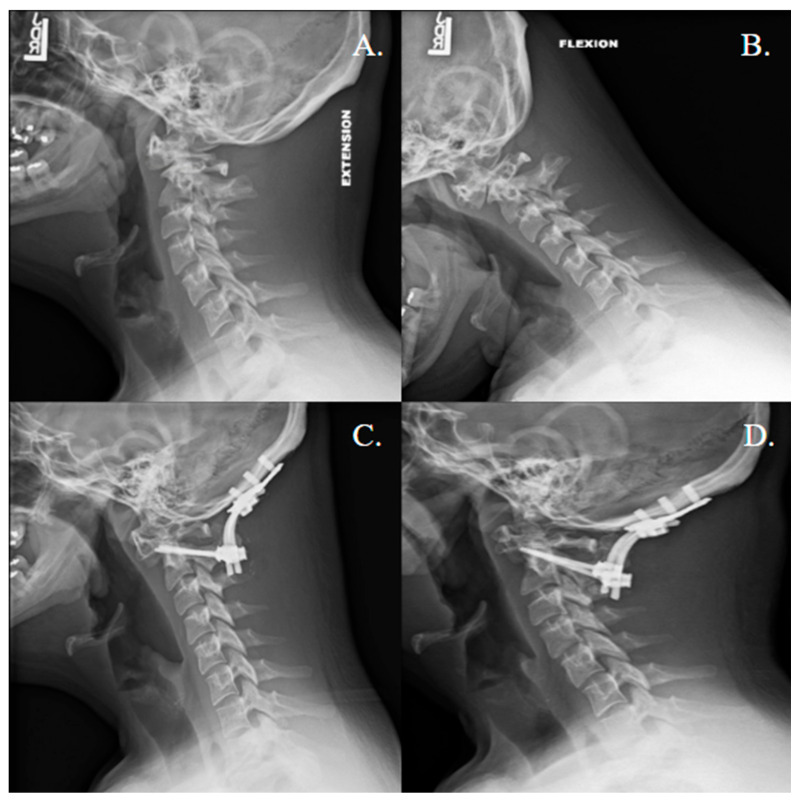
Atlantoaxial instability in Down syndrome: dynamic instability, construct, and follow-up. (A) Pre-operative lateral extension radiograph with ADI measuring 3 mm. (B) Pre-operative lateral flexion radiograph with anterior ADI measuring 6 mm, demonstrating dynamic C1–2 instability. (C) Immediate post-operative lateral radiograph demonstrating C1–2 instrumented fusion with appropriate hardware position and reduction in the ADI. (D) One-year post-operative lateral radiograph showing maintained reduction, no hardware lucency, consistent with fusion. Abbreviations: ADI: anterior antlanto dental interval; LDR: low dose reconstruction.
